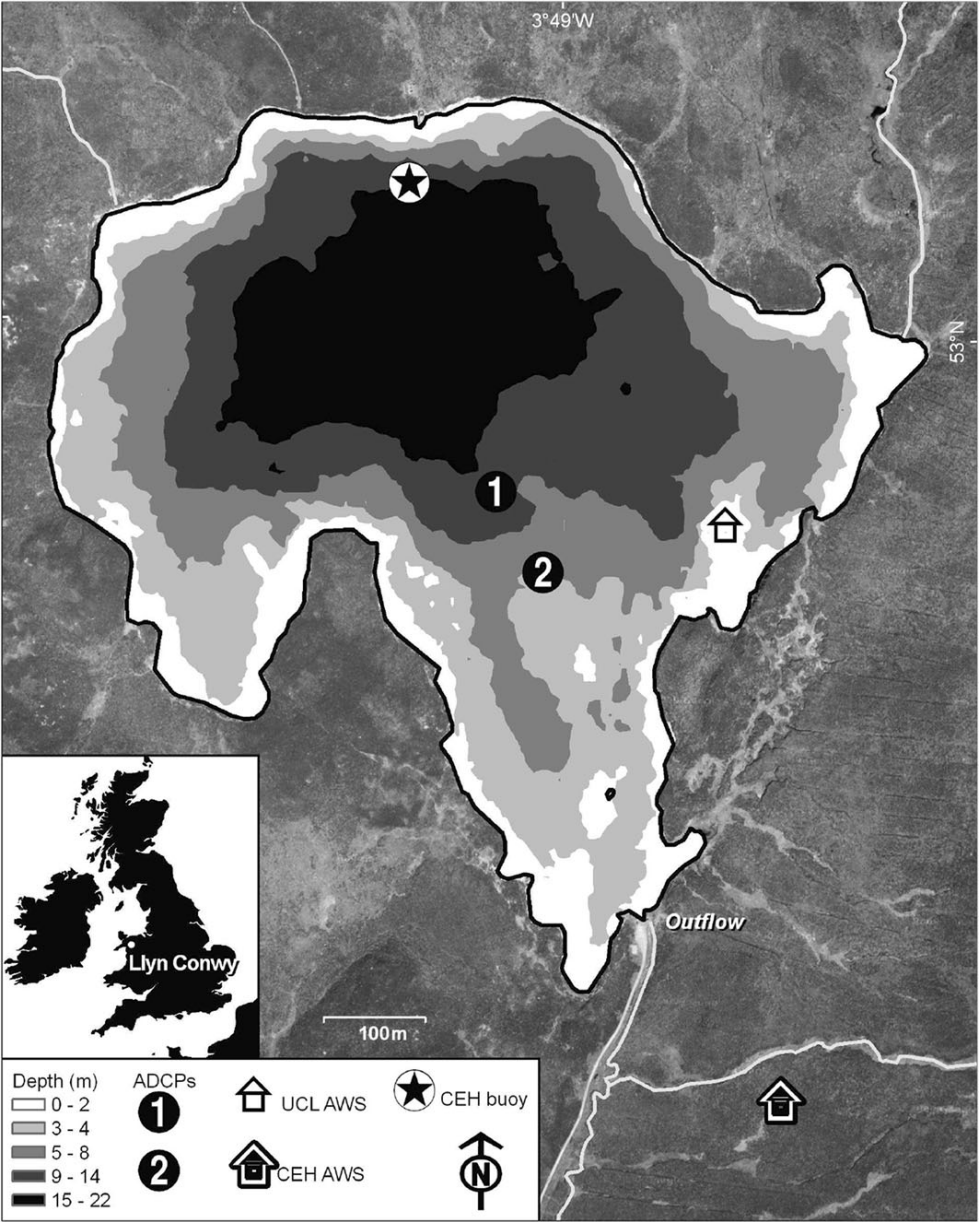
Llyn Conwy location, bathymetry (depth contours in m) and data acquisition locations. Automatic Weather Stations (AWS) recorded wind speed and direction, air temperature and pressure, and relative humidity. CEH data buoy includes an AWS and also a sensor string to measure vertical variation in water temperature. Acoustic Doppler Current Profilers (ADCPs) are upward-looking bottom deployments. See text for further information
